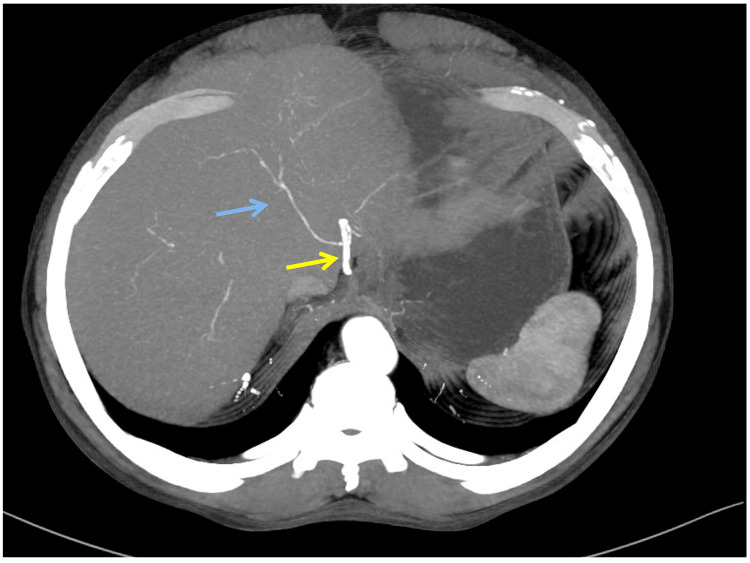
Contrast enhanced CT of the abdomen shows a replacement left hepatic artery (blue arrow) from the left gastric artery (yellow arrow).
